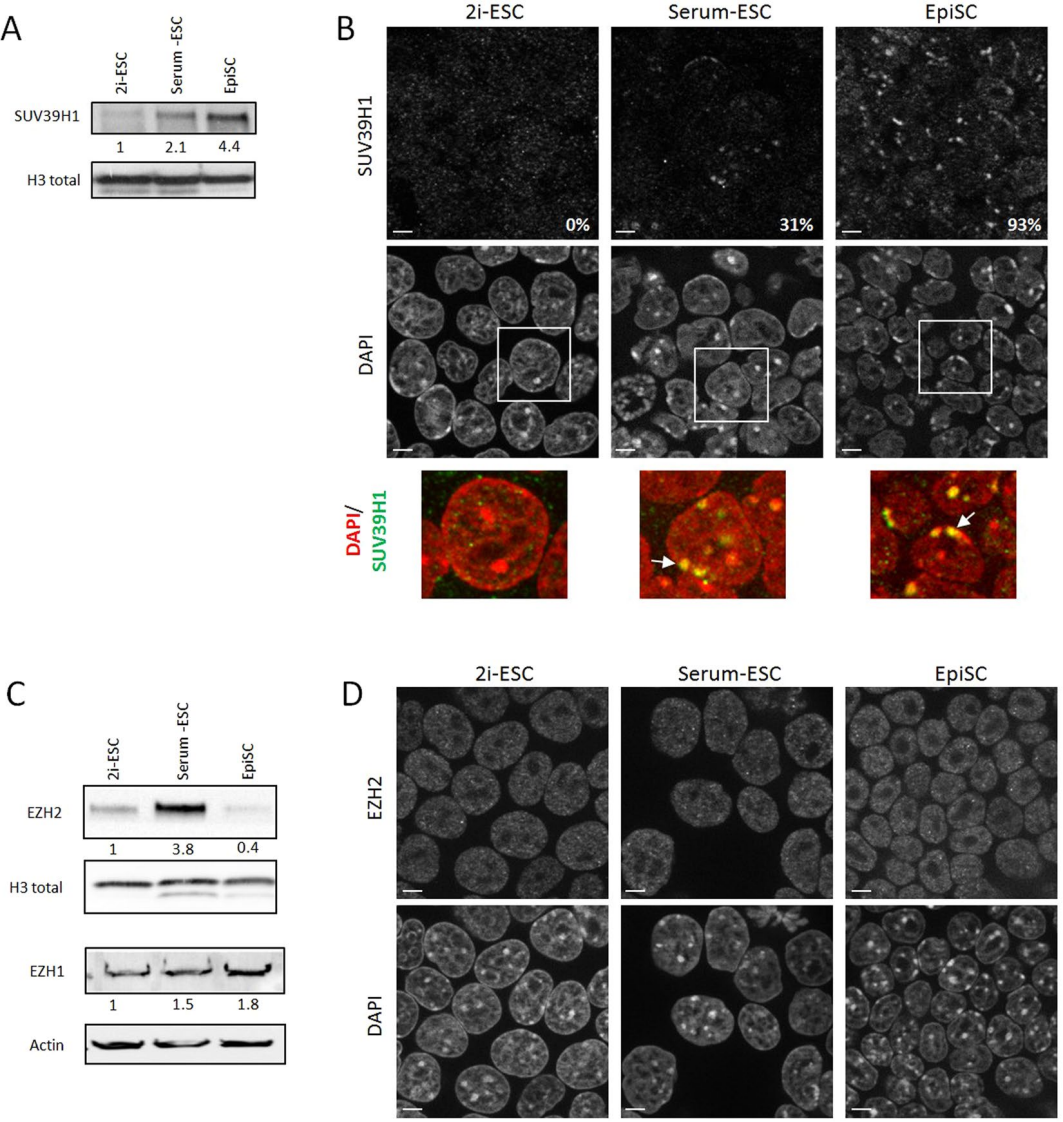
Expression and pattern of epigenetic modifiers (A) Western-blots for SUV39H1 and H3. (B) Single plan images of the 3 cell types immunolabelled with antibody against SUV39H1 (scale bars represent 5 µm). A representative enlarged nuclei (white squares, lower panel, DNA in red and SUV39H1 in green) and the percentage of cells in the population displaying DAPI clusters enriched with SUV39H1 (white arrows) are indicated for each cell type. (C) Western-blots for PRC2 enzymes, EZH2 and EZH1, and H3 or Actin. (D) Single plan images of the 3 cell types immunolabelled with antibody against EZH2 (scale bars represent 5 µm).
